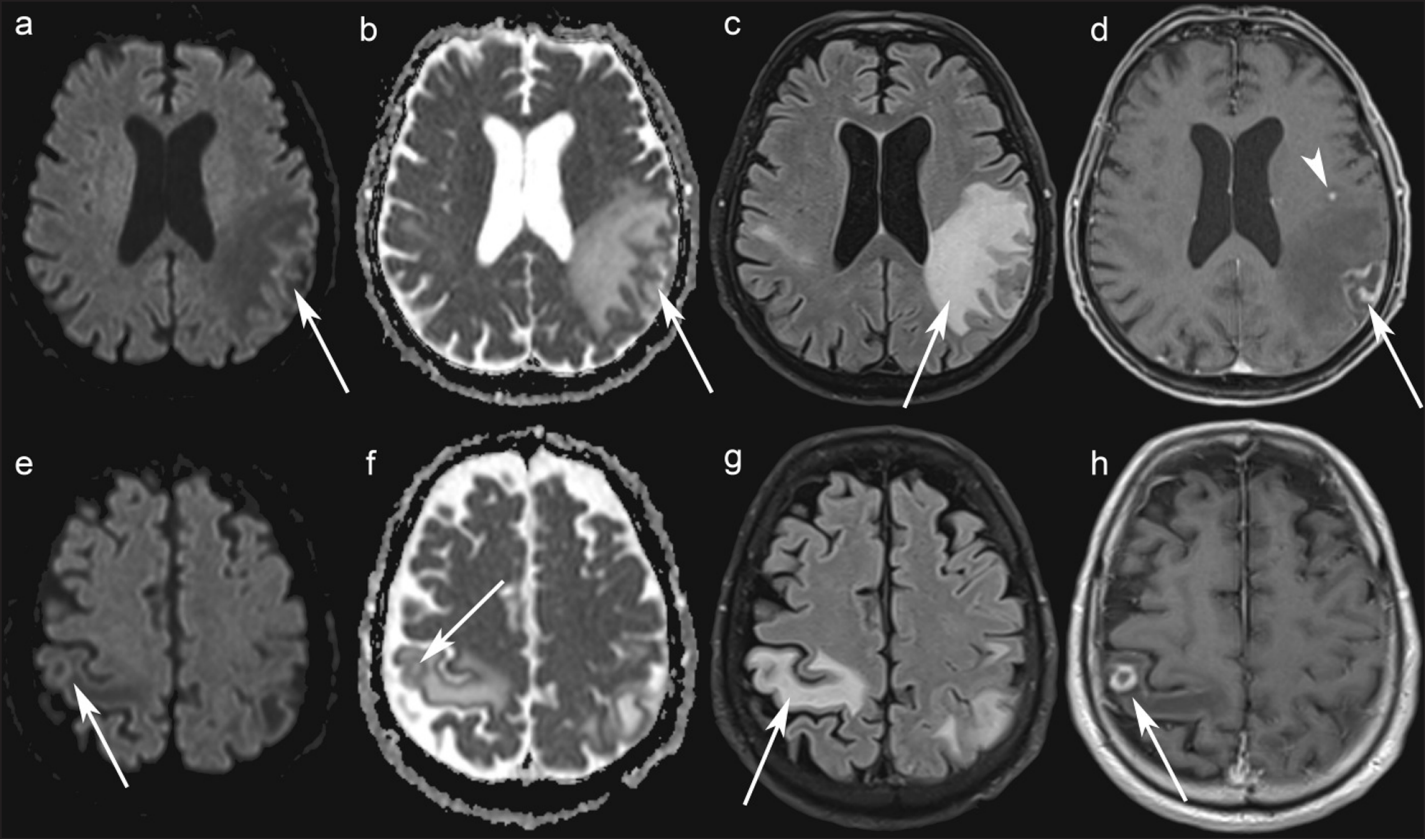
Brain MRI. Axial Diffusion-Weighted Imaging (b = 1500 s/mm) show no increased signal in the left and right parietal lobe lesions (a, e) while axial ADC maps show a greater signal than that of the unaffected white matter (b, f) (arrows). Axial FLAIR images show edema around the lesions (c, g). Axial contrast-enhanced T1-WI shows rim-enhancing lesions in the left and the right parietal lobes (arrows) (d, h), and one punctate lesion in the left frontal lobe (arrowhead) (h).
